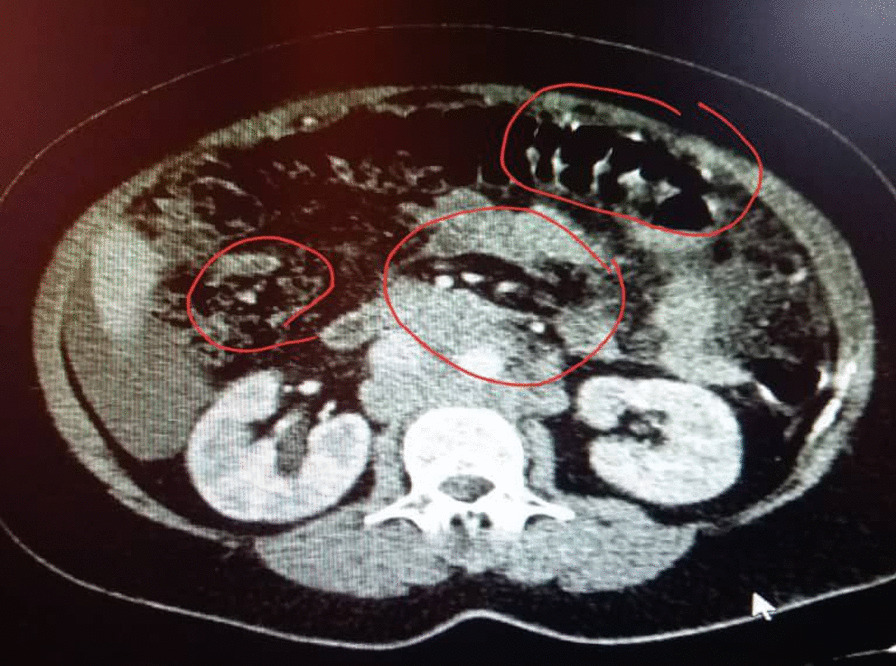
The computed tomography showed also enlargement lymph nodes around the abdominal aortic and right iliac vessels with signs of mesenteric metastases and moderate ascites. The red circles reveal the lymphadenopathy
